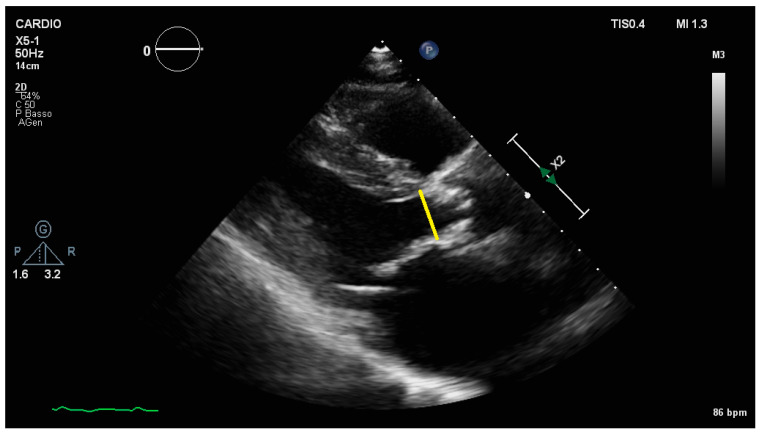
Measurement of the diameter of the Left ventricular outflow (yellow line) from the parasternal long-axis view, during systole, directly below the base of the aortic leaflets, and from the internal surfaces of the interventricular septum and the posterior aortic wall.
